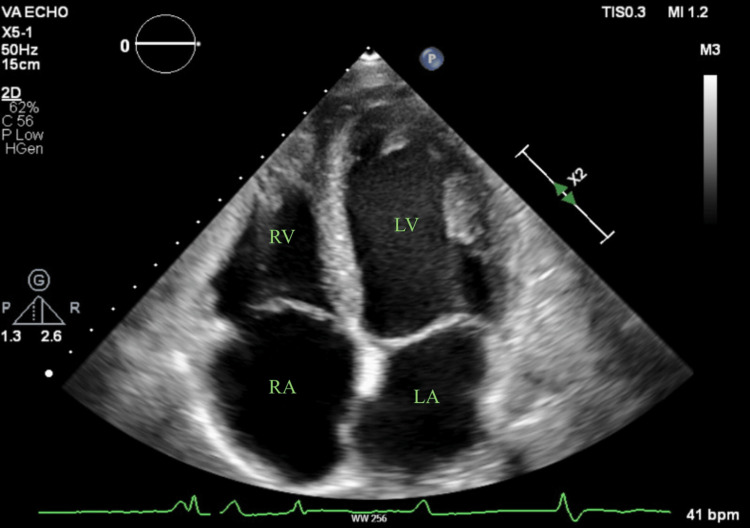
Apical four-chamber view noting biatrial enlargement, bright myocardium, and dilated LV RA, right atrium; RV, right ventricle; LA, left atrium; LV, left ventricle
